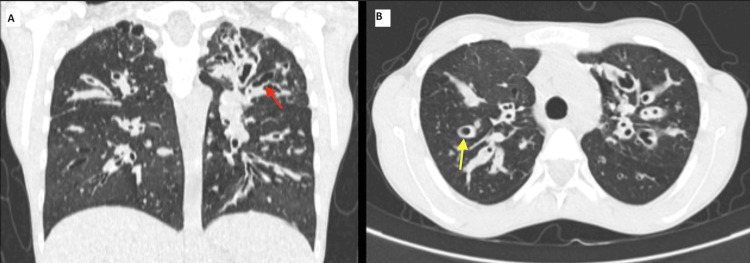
A computed tomography chest showing tubular and cystic bronchiectasis Red arrow: tubular bronchiectasis; yellow arrow: cystic bronchiectasis
